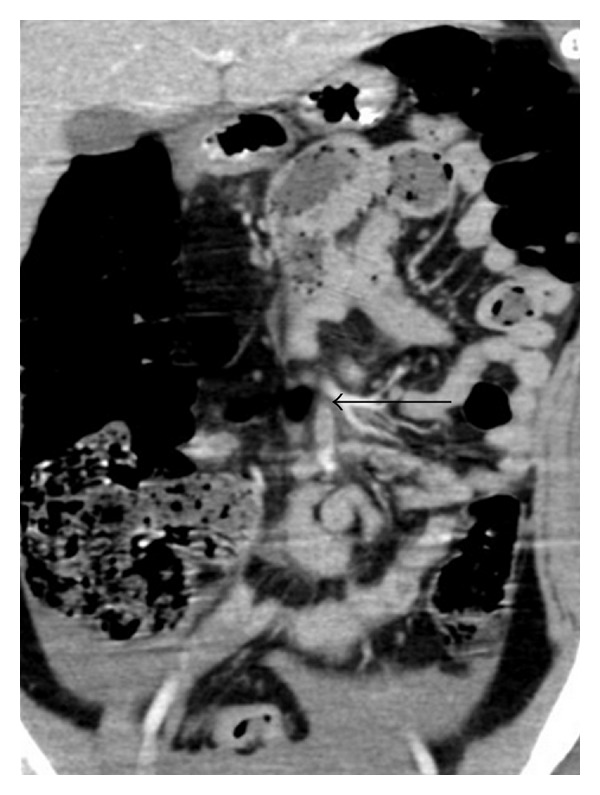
Coronal view arterial phase CT abdomen. Filling defect in main SMV (long arrow) and large amount of intraperitoneal blood.
